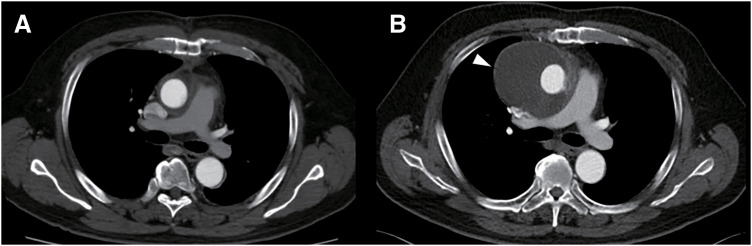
CT findings. (A) CT scan obtained 1 month postoperatively revealed no perigraft fluid collection. (B) CT scan obtained 5 years postoperatively revealed a low-density area (white arrow) suggestive of perigraft fluid collection. CT: computed tomography
